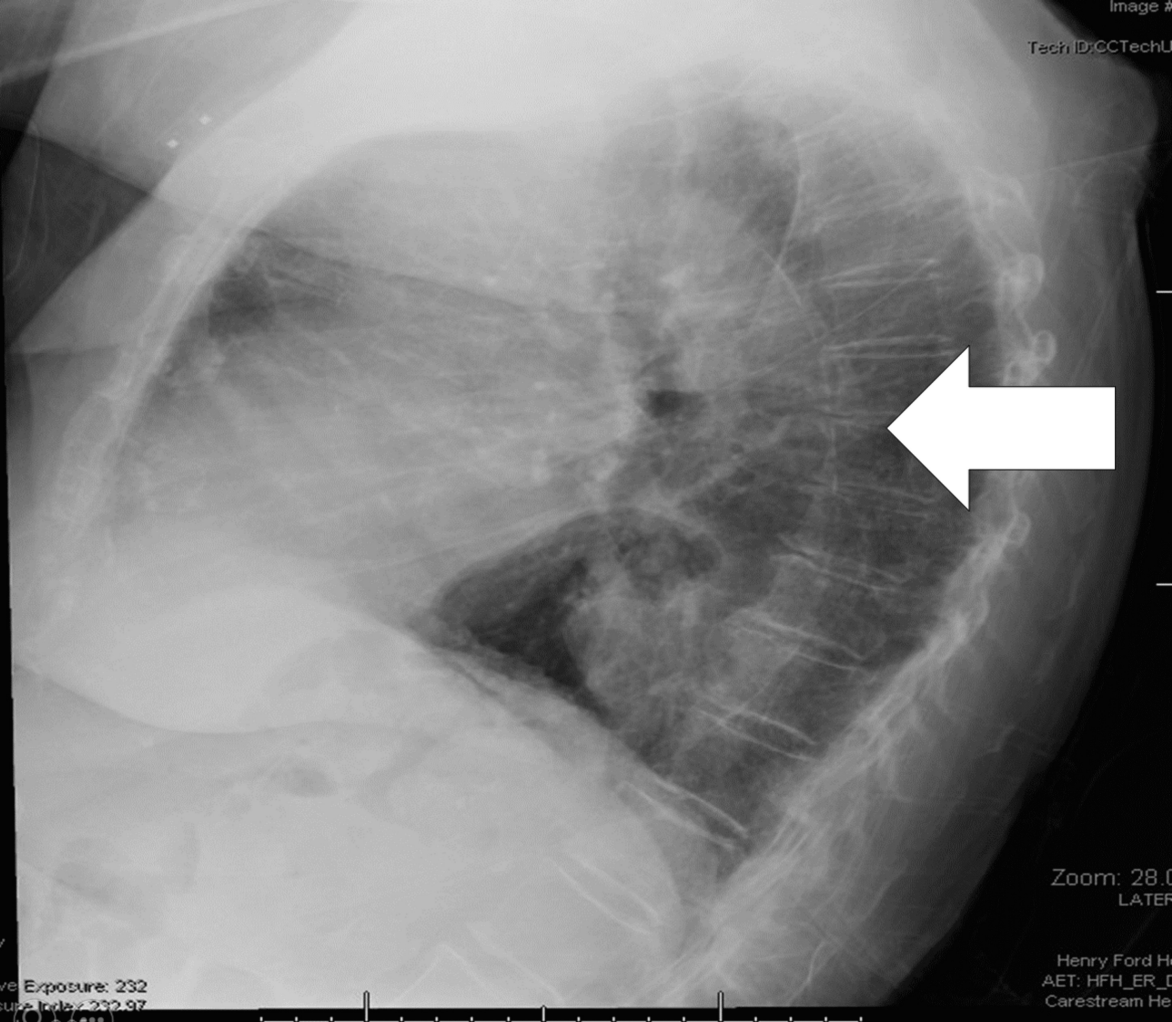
Lateral radiograph of moderately sized hiatal hernia from three months prior to presentation
